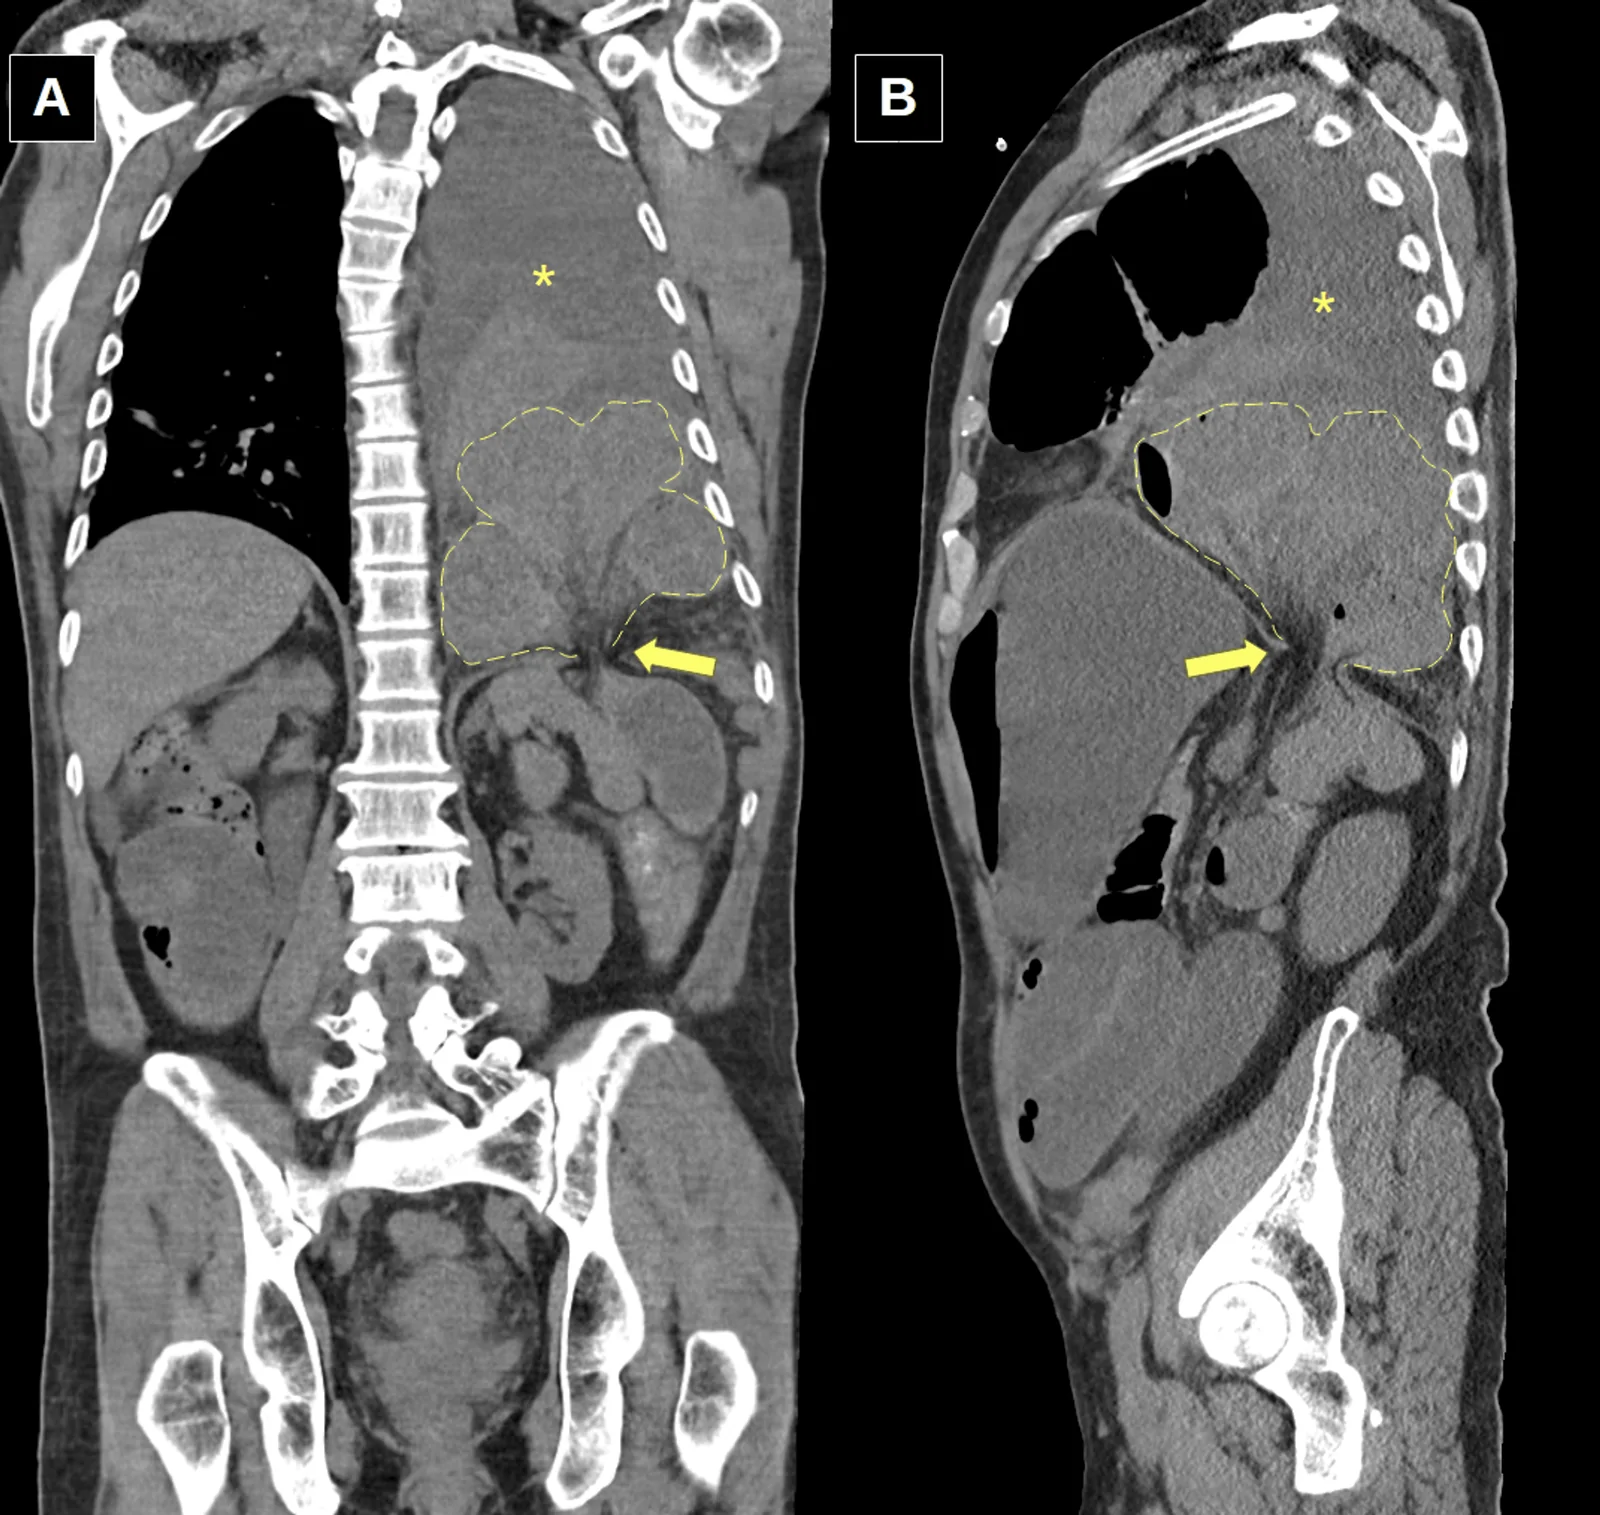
Multiplanar CT images demonstrating herniation of the small bowel through a left-sided diaphragmatic defect. (A) Coronal view and (B) sagittal view. Images show herniated bowel loops (dashed lines) traversing the defect. The asterisk (*) marks indeterminate structures, possibly herniated omentum versus collapsed lung, not definitively distinguishable on these views.
